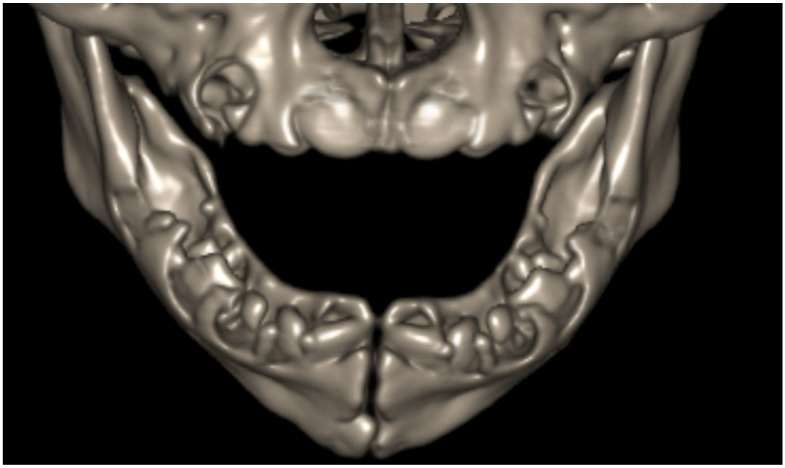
Three-dimensional CT reconstruction of the dental arches in a neonate revealing the numerous bony crypts in which multiple primary teeth are developing.
